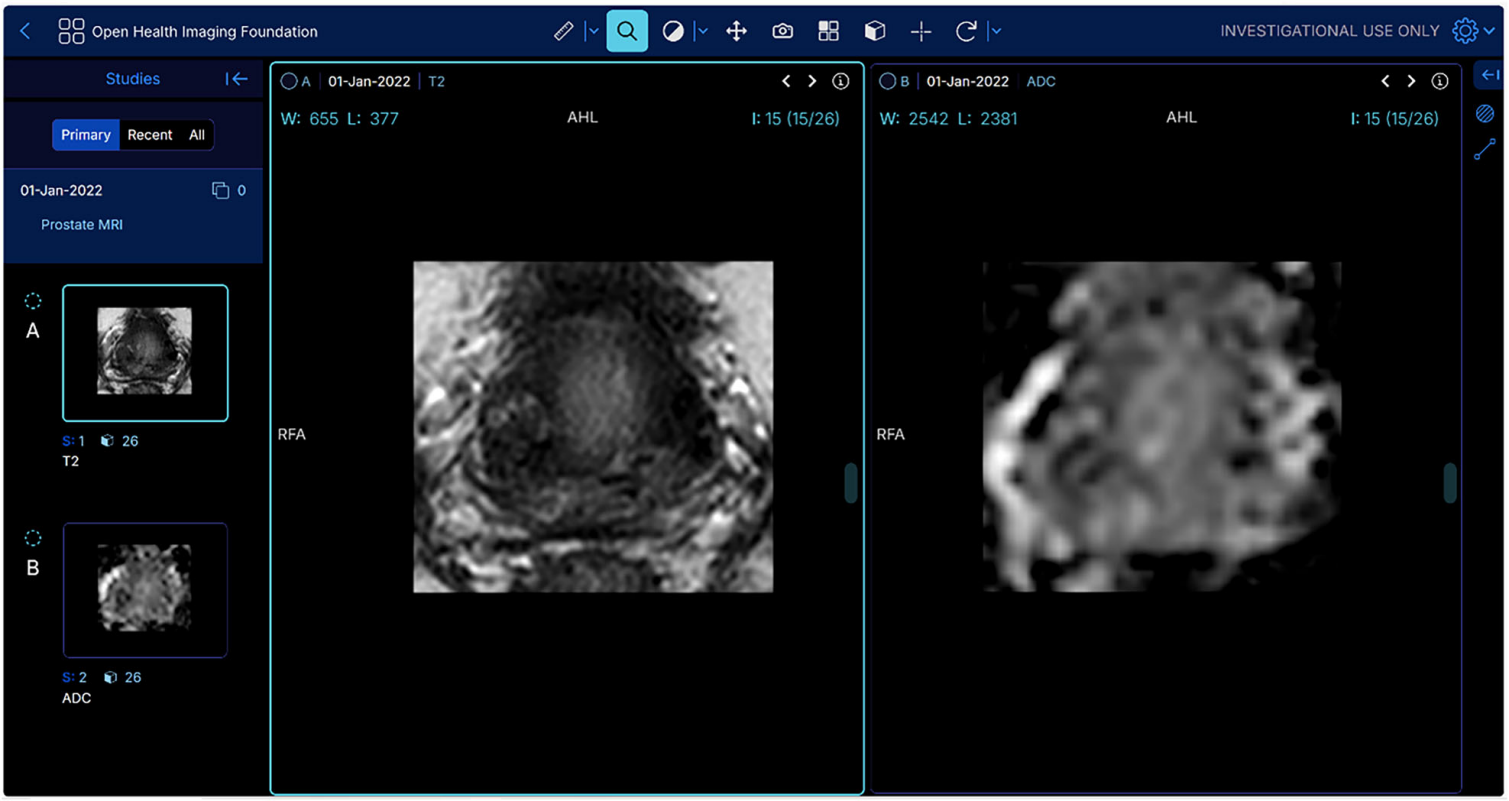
Prostate-focused T2W MRI and ADC maps. The readers evaluated 74 pairs of T2W MRI and ADC maps using side-by-side visualization in the displayed format. They had the option to adjust the windowing settings and could reset the view to explore different viewing preferences if needed. A zoom option was also available
